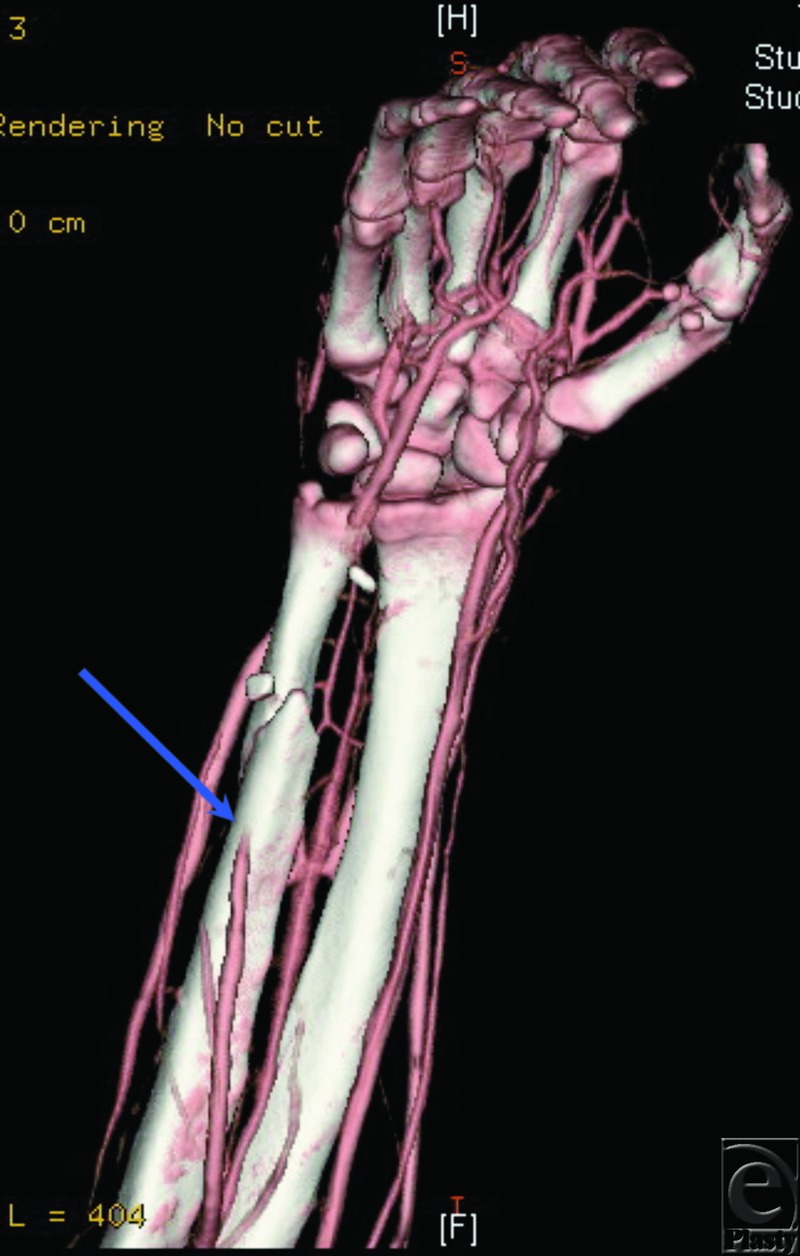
CTA in reconstruction of the upper limb. CTA of the right upper extremity demonstrating occlusion of the ulnar artery in the distal forearm at the level of the fracture site. The hand is supplied through the radial artery and a complete palmar arch.
